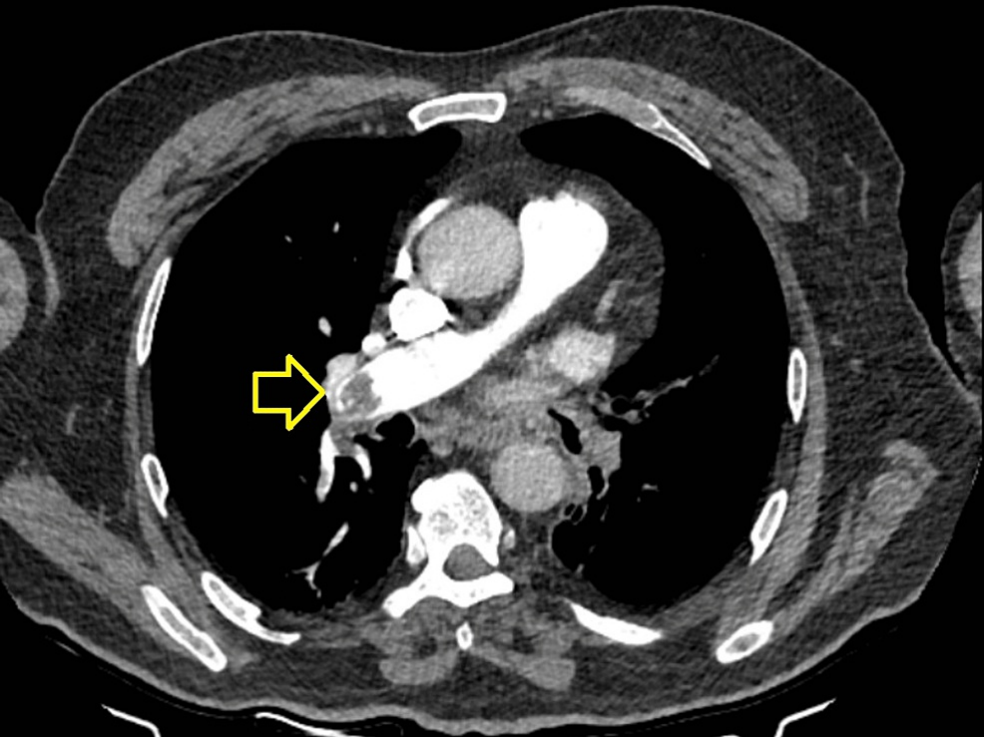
Pulmonary embolism CT view
